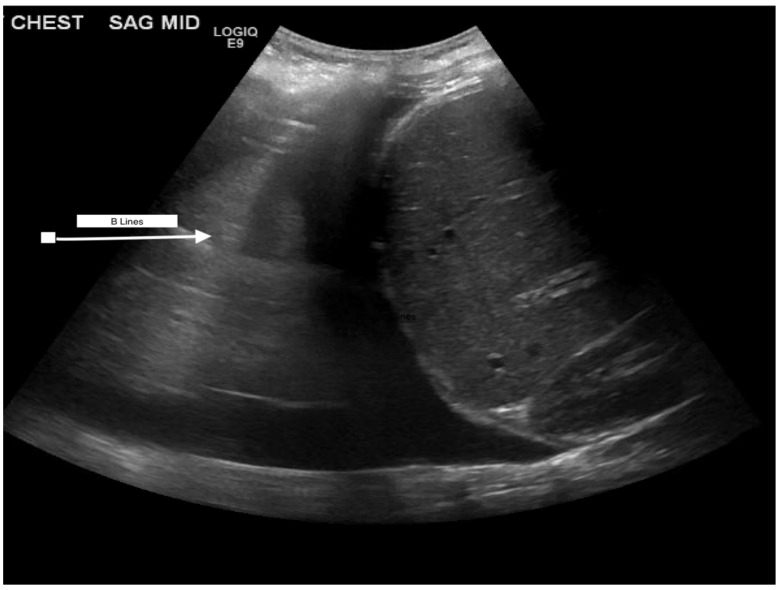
B-lines in thoracic ultrasound in a child with pulmonary edema. The ultrasound appearance is of a vertical, discrete, hyperechogenic line that arises from the pleural line and extends to the bottom of the screen, moving synchronously with respiration.
